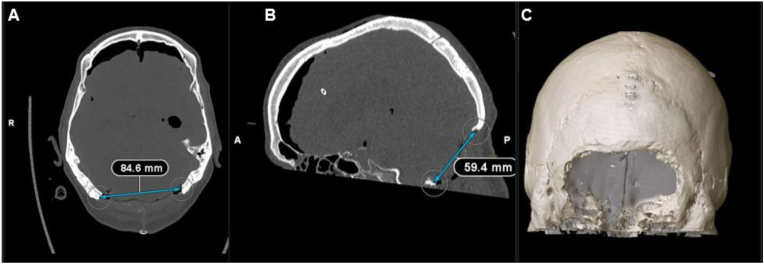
Measurements of craniectomy size in postoperative CT scans. A Determination of maximum lateral diameter on axial CT scan. B 3D reconstruction of postoperative CT scan demonstrating craniectomy size as an ellipse. C Determination of maximum craniocaudal diameter on sagittal CT scan.
